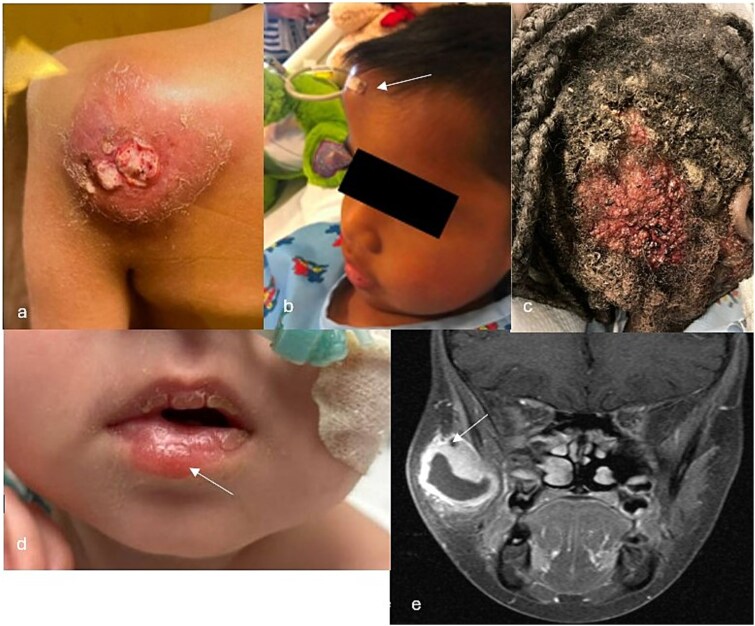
Photo collage of interesting clinical presentations, these represent patient sites of infection that all grew *Coccidioides* in culture obtained during biopsy or debridement. (a) Ulcerated mass on the supraspinatus muscle with scapular involvement. (b) Right frontal bone protruding lesion (MRI previously published).^19^ (c) Parietooccipital scalp lesion that invaded underlying bone (previously published).^20^ (d) Cutaneous lip nodule. (e) Right masseter muscle abscess with bony destruction of the right zygomatic arch
